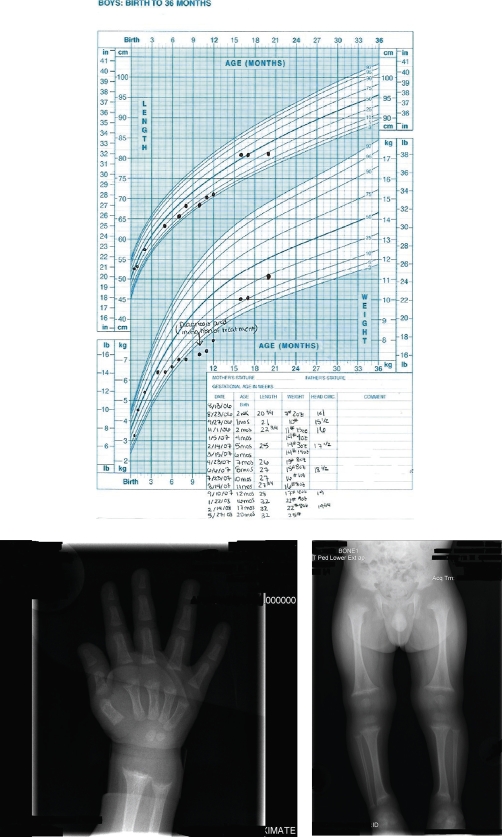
Growth Chart: Growth chart from birth to 20 months showing failure of appropriate growth and then his catch up growth after appropriate treatment. Radiograph Wrist/Hand: Metaphyseal fraying and cupping of distal radius and ulna and the distal aspects of the second through fifth metacarpals. Skeletal survey: Metaphyseal fraying and cupping involving bilateral distal femurs, bilateral proximal and distal tibiae and fibulae, bilateral proximal and distal humeri.
